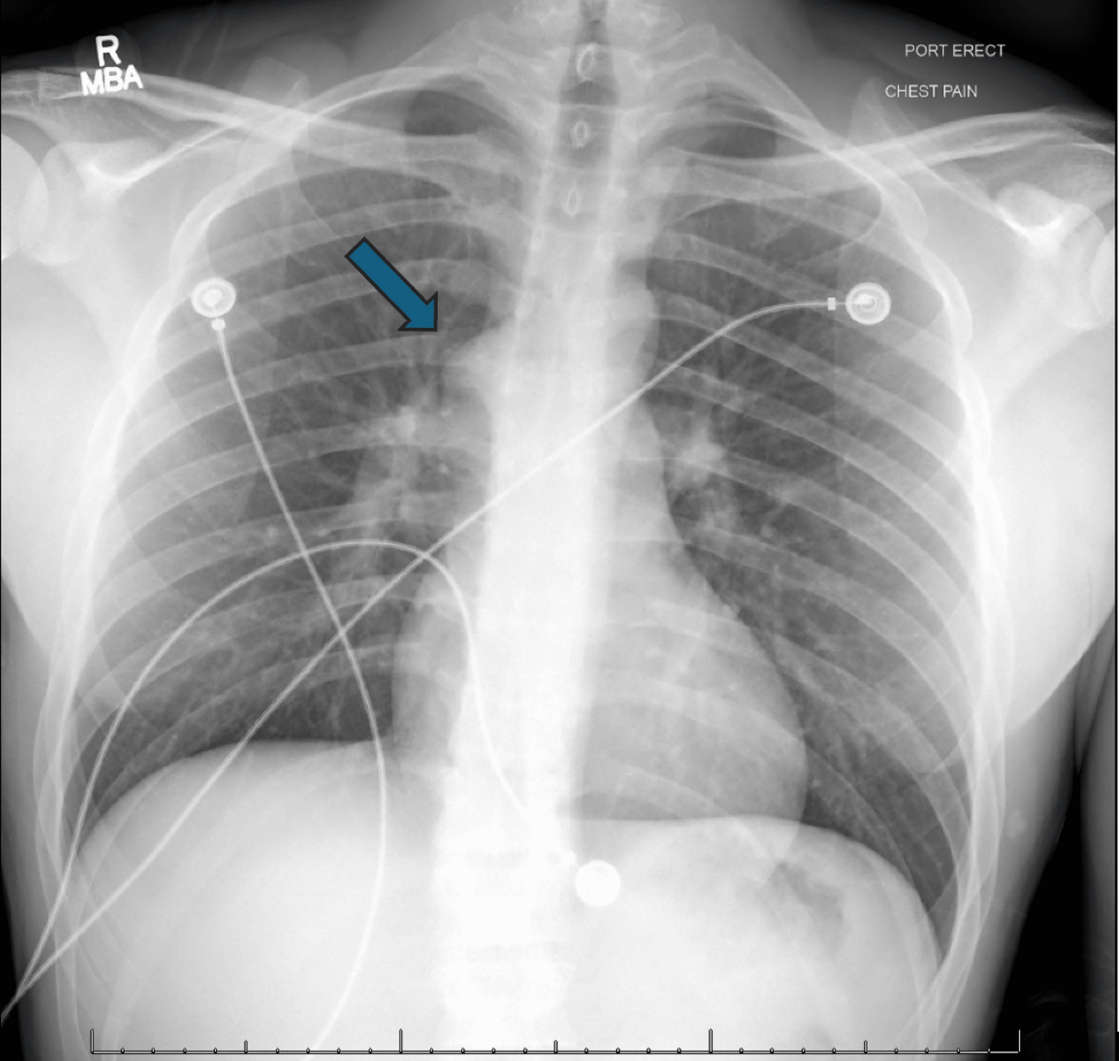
Chest x-ray. The blue arrow points at prominence in the azygous region. This prominence corresponds to the distended azygos vein, which was compensating for the interrupted IVC, serving as the primary route for venous return from the lower body to the superior vena cava.
